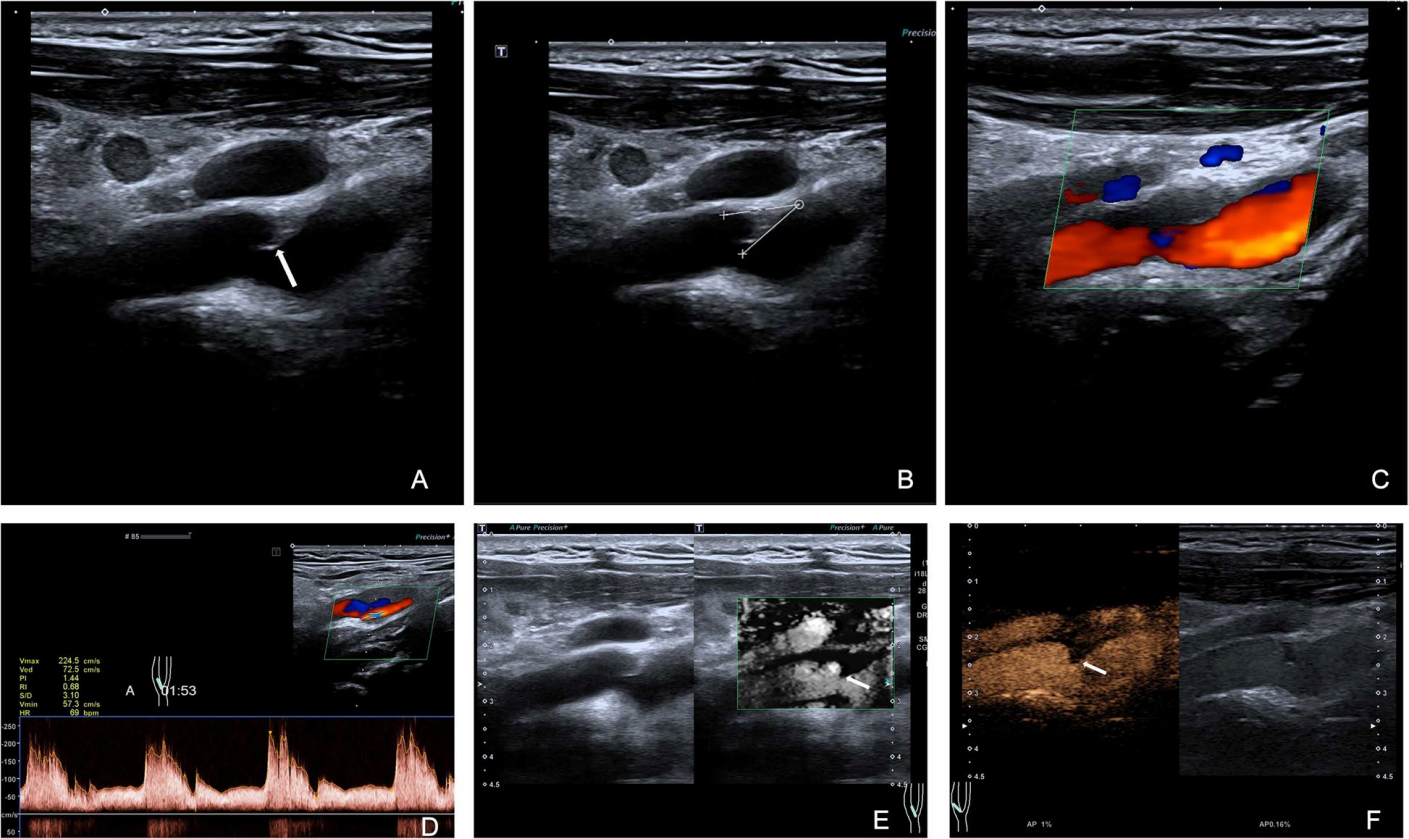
Multimodal ultrasound features of a carotid web (white arrow) locates on the anterior wall of the right carotid artery bifurcation are presented. A An isoechoic shelf-like structure protrudes into the lumen. B The carotid web angle (31.3°) is measured using computer-aided software. C Color doppler ultrasound reveals swirling blood around the web. D The carotid web causes moderate luminal stenosis. E Superb microvascular imaging reveals a triangular endoluminal defect on the longitudinal plane. F Contrast-enhanced ultrasound shows no enhancement of the web
